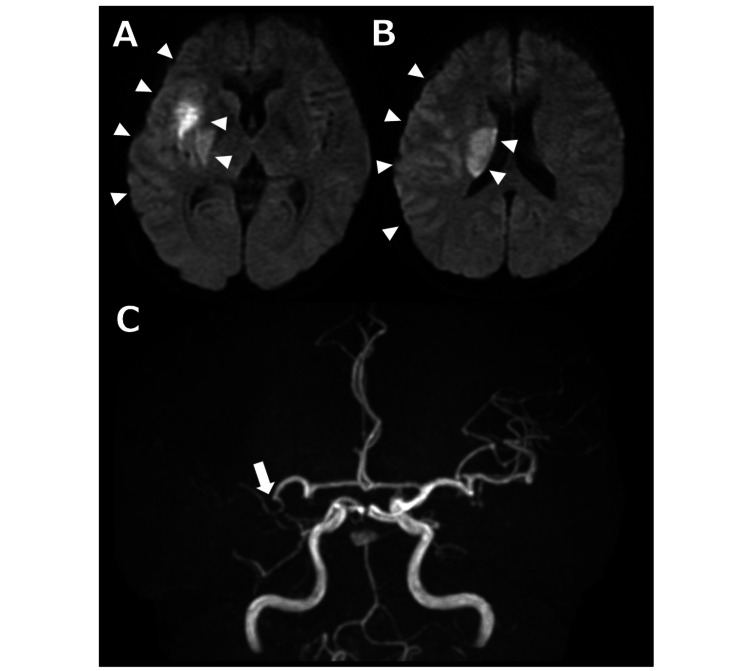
Brain MRI and magnetic resonance angiography on admission. Brain MRI (A, B) reveals high signal intensities on diffusion-weighted imaging in the territory of the right middle cerebral artery (arrowheads). Magnetic resonance angiography (C) demonstrates occlusion of the right middle cerebral artery at the distal M1 (horizontal) segment (arrow).
